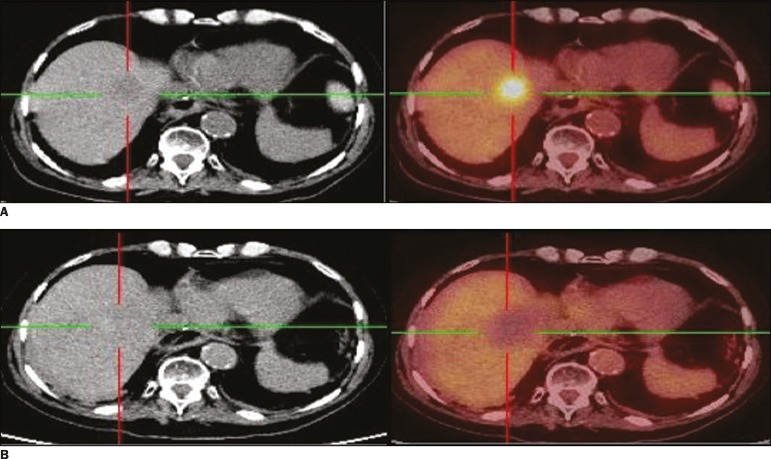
A: Baseline ^18^F-FDG PET/CT images of a liver
